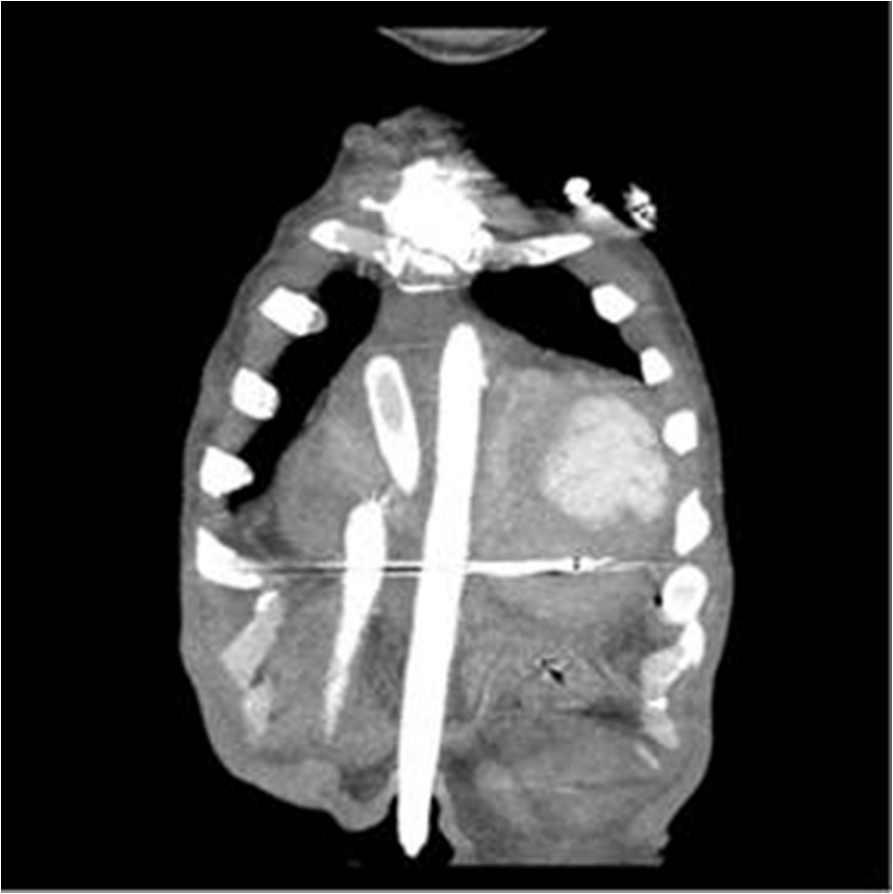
Appearance of the cannulas on CT angiography.
